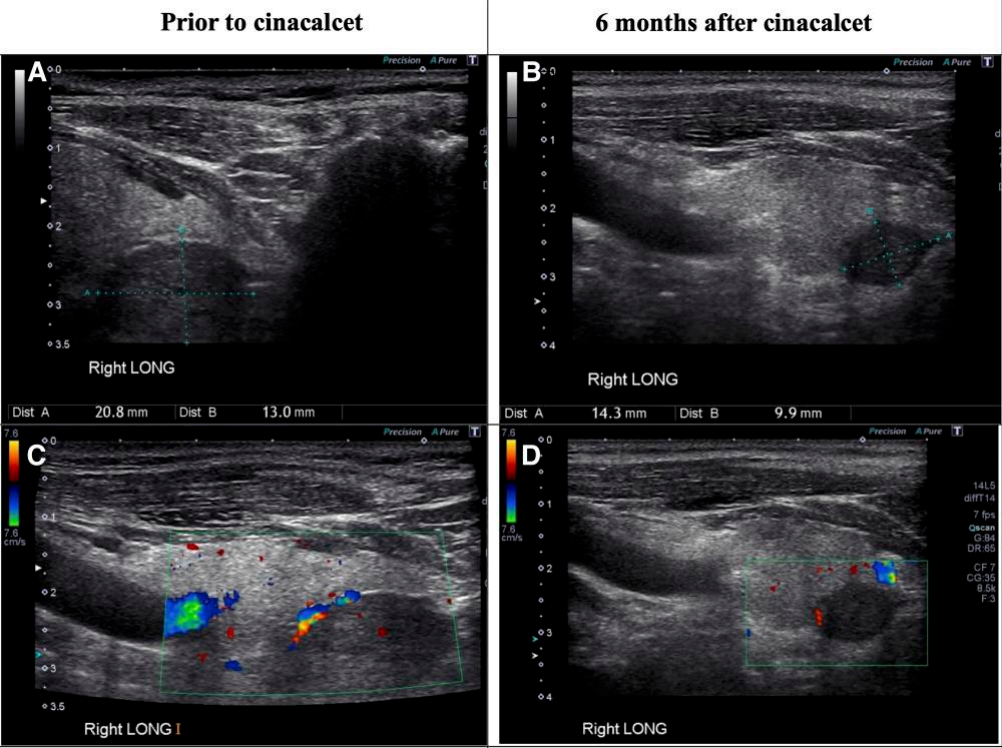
Ultrasound image of presumed parathyroid adenoma prior to initiating cinacalcet and after 6 months of treatment with cinacalcet. (A) 2.3 × 1.7 × 3.0 cm mass posterior to right lobe prior to cinacalcet. (B) 1.4 × 1.0 × 1.1 cm mass posterior to right lobe 6 months after cinacalcet. (C) Hypoechoic with clear borders and feeding vessel. (D) Doppler images of the same mass.
